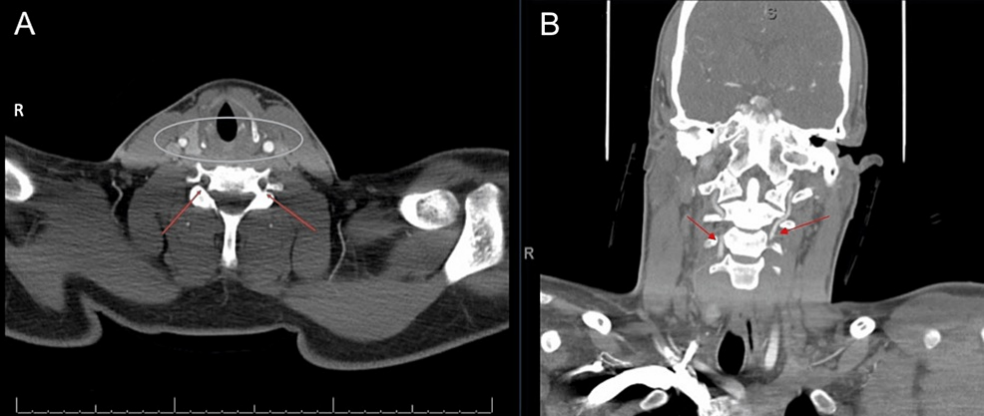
(A) CT angiography neck with contrast showing loss of contrast opacification of the bilateral vertebral arteries at the V1 (preforaminal)-V2 (foraminal) junction at the level of C5 consistent with bilateral vertebral artery dissection (arrows). No traumatic injury to the carotid arteries was seen (circle). (B) CT angiography neck with contrast showing reconstitution and normal contrast opacification and vessel caliber at approximately the C3 levels (arrows). CT, Computed Tomography
